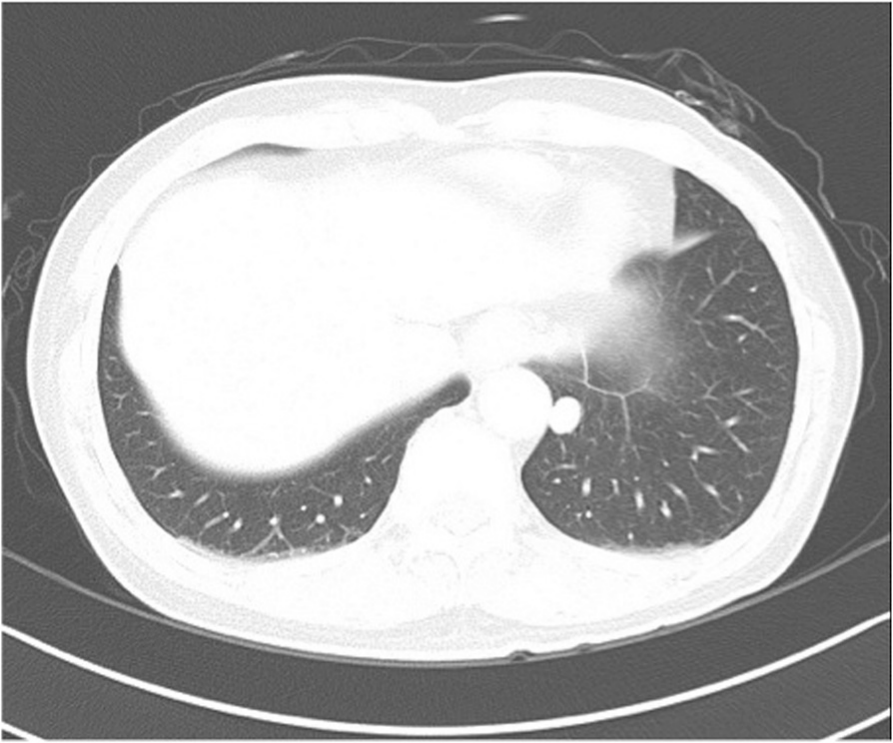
Chest computed tomography (CT) of case 1 shows a nodular shadow in the left S10
